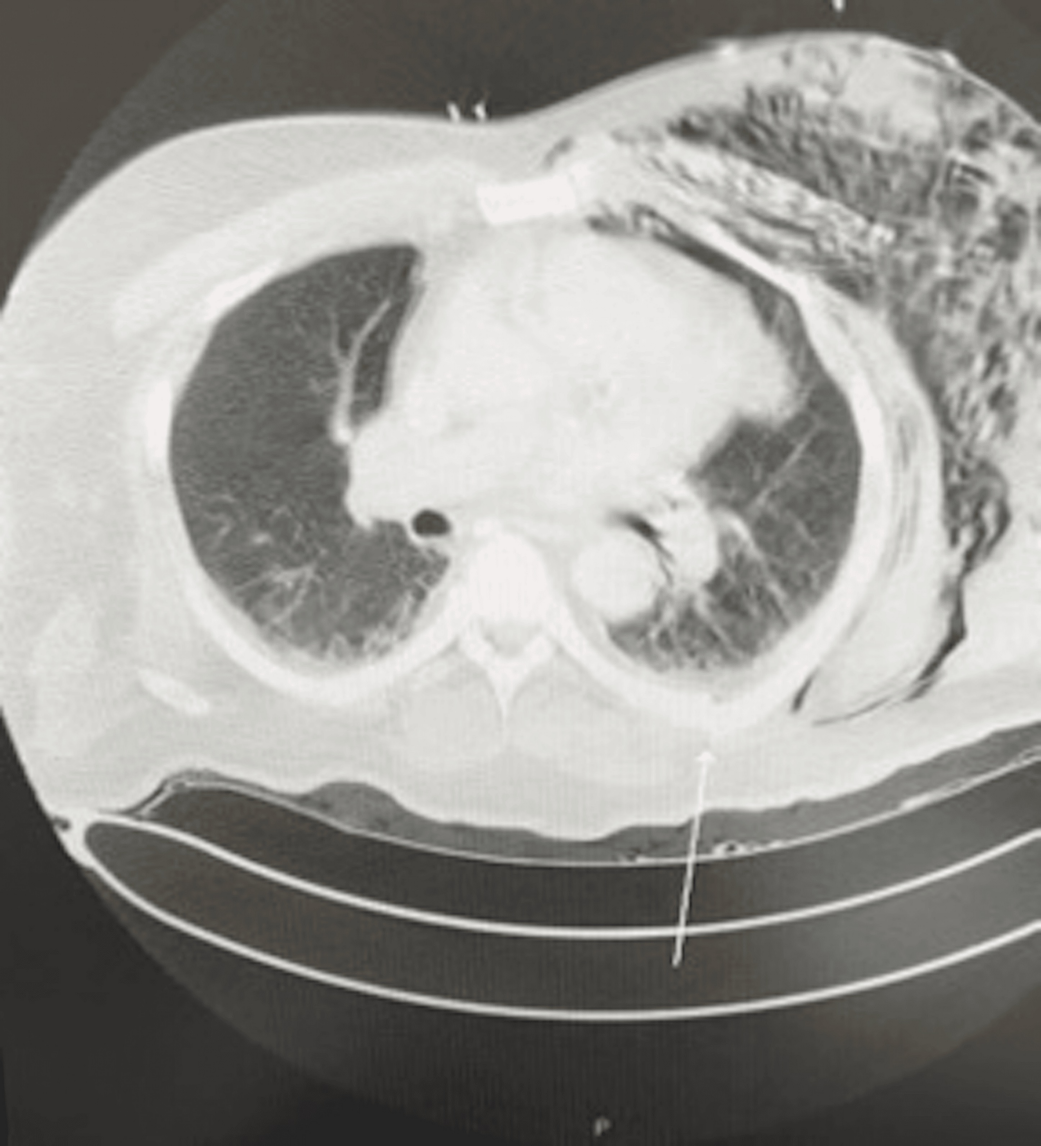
Computed tomography chest imaging demonstrating large volume subcutaneous emphysema, trace left hemopneumothorax, and posterior medial fracture of the sixth rib (white arrow).
